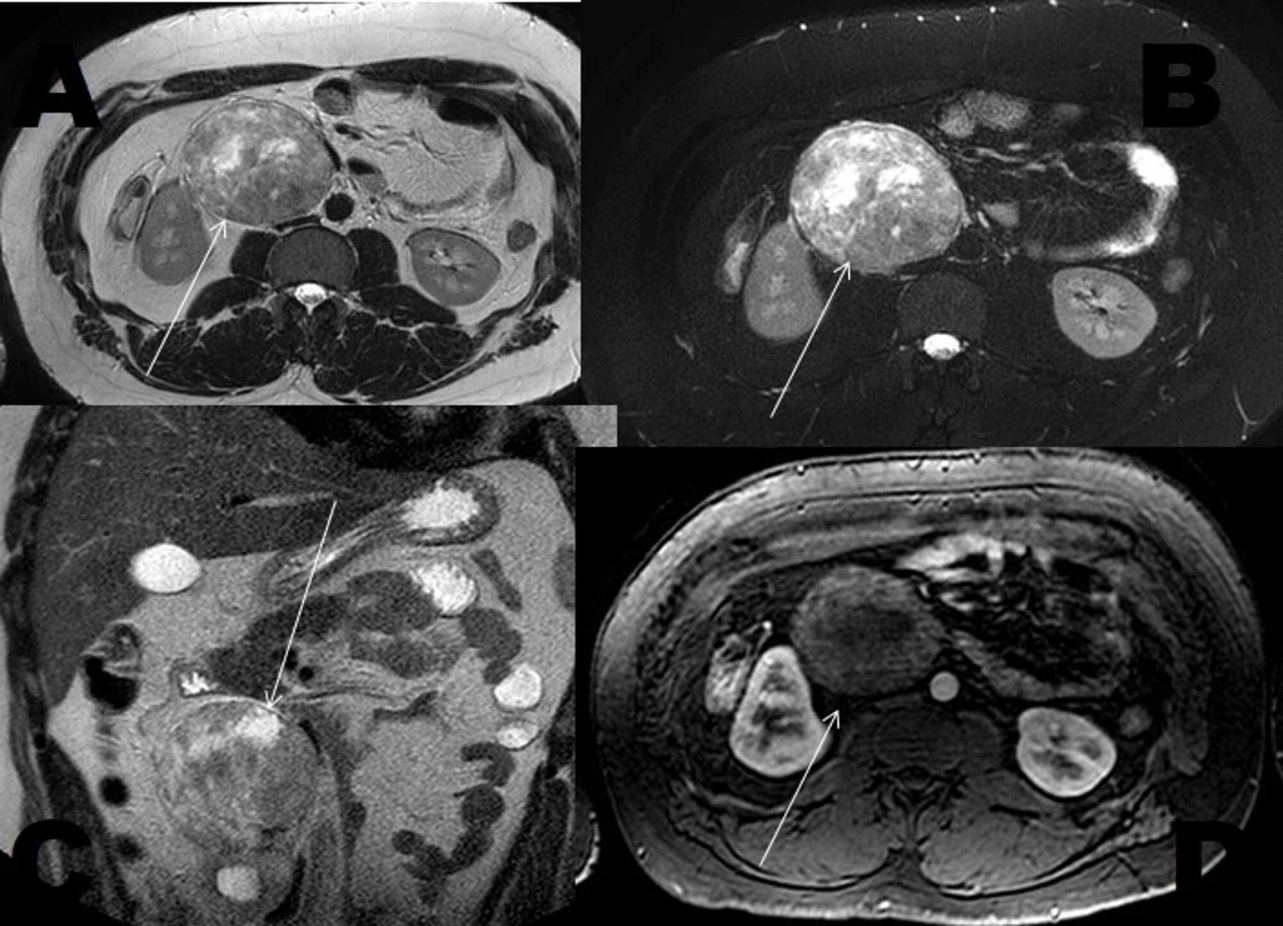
Abdominal MRI: Axial sections in T2 sequence (A), T2 with fat saturation (B), T2 coronal sections (C), and axial T1 sections with fat saturation and gadolinium contrast (D) showing a large retroperitoneal mass (arrows), well-circumscribed, hyperintense in T2 sequence, heterogeneous with cystic areas not enhanced by contrast.
